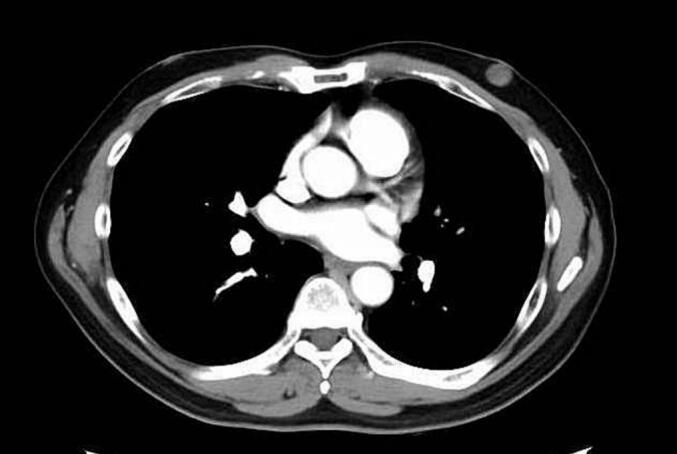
A contrast-enhanced computed tomography scan revealed a mass with a clear border directly below the nipple.
